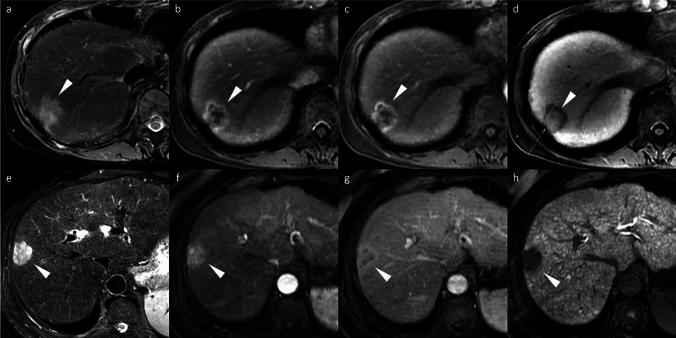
Two types of mass-forming intrahepatic cholangiocarcinoma (ICC) in a 63-year-old woman (a–d) and a 69-year-old man with hepatitis C virus-related cirrhosis (e–h). In the 63-year-old woman, ICC at the posterior segment (arrowheads) shows hyperintensity on fat-suppressed T2-weighted imaging (a), target appearance in the arterial phase (b), and portal venous phase (c), as well as intermediate signal intensity or target appearance (arrow) in the hepatobiliary phase (d), a pattern referred to as “cloud enhancement.” In the 69-year-old man, ICC at the anterior segment (arrowheads) shows hyperintensity on fat-suppressed T2-weighted imaging (e), arterial phase hyperenhancement (f) with portal venous washout (g), and hypointensity in the hepatobiliary phase (h). Differentiation from hepatocellular carcinoma is challenging based on imaging features
